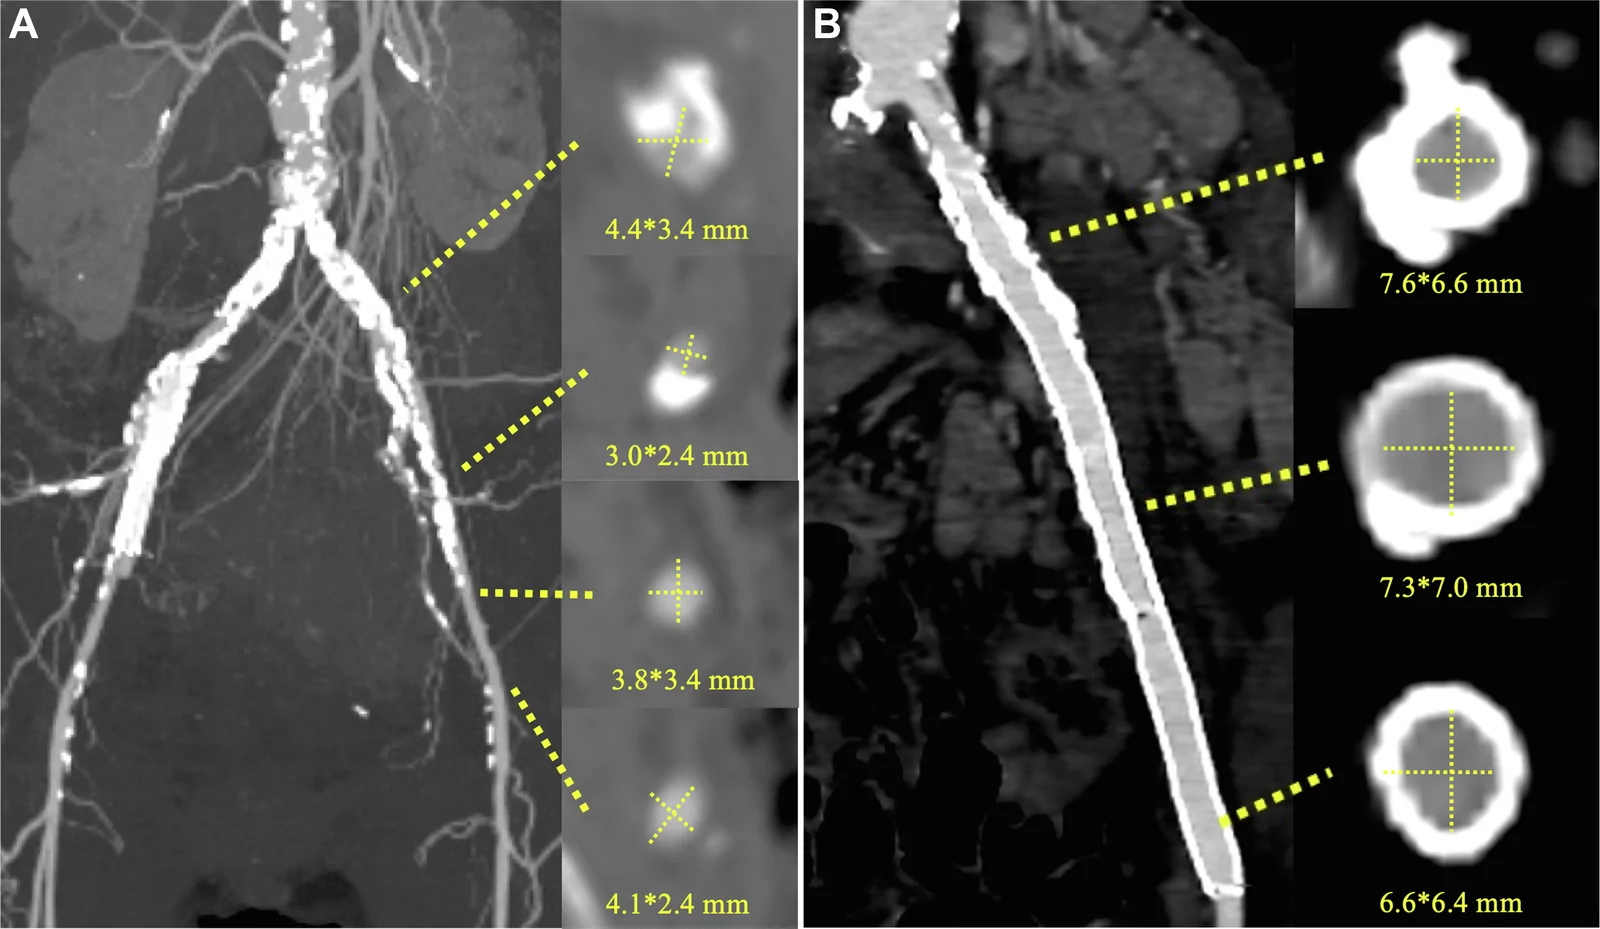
CT Angiography of the Left Access Artery Before and After the Internal Endoconduit (A) MIP of the preoperative CT angiogram reveals heavily calcified and severely stenotic iliac and common femoral arteries. The reconstructed cross-sectional images perpendicular to the center line of the left iliofemoral artery demonstrate markedly reduced luminal diameters, ranging from 2.4 to 4.4 mm. (B) MIP of the postoperative CT angiogram demonstrates a stent graft–supported endoconduit along the left iliac and common femoral arteries. Cross-sectional images of the respective portions show marked luminal expansion, with diameters ranging from 6.4 to 7.6 mm, which most likely indicated rupture of the native iliac artery after stent graft deployment and balloon angioplasty. CT = computed tomography; MIP = maximum intensity projection.
